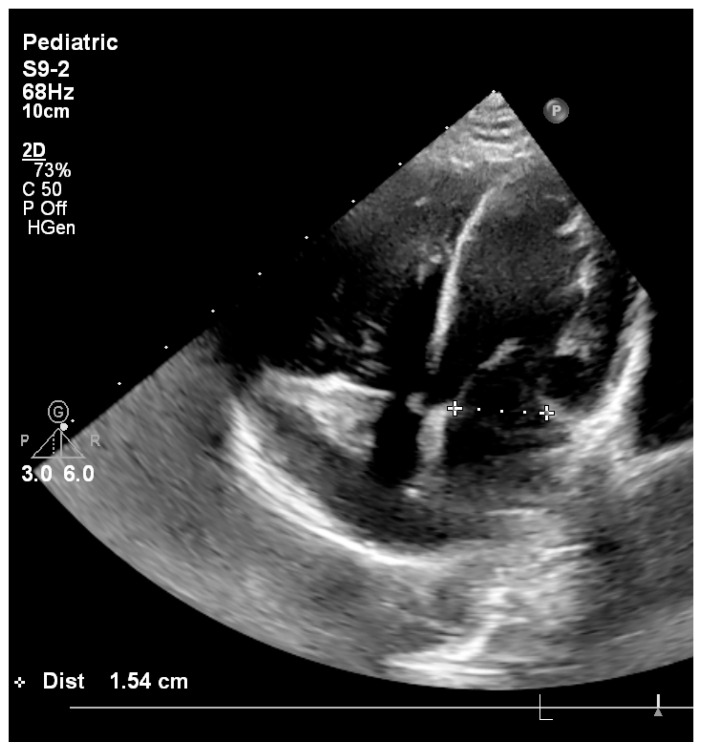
Follow-up transthoracic echocardiography demonstrating normalization of the left-sided cardiac chambers, with a mitral valve dimensions of 1.54 cm, 2 years after biventricular repair.
